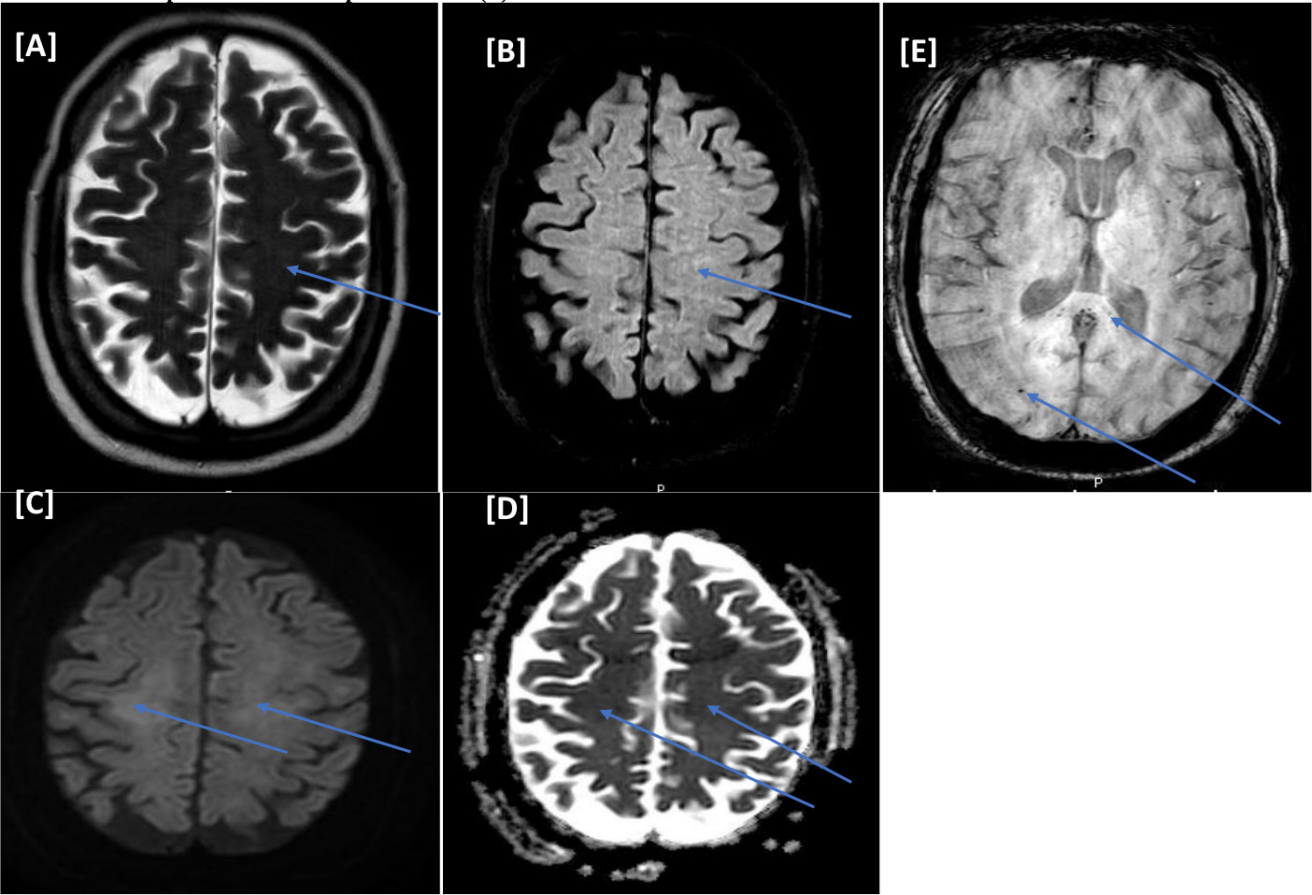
(Case III) [A–E images] Magnetic resonance imaging of the head. Bilateral centra semiovale white matter faint bright signal intensities on T1WI/FLAIR (A and B) with corresponding faint restriction on diffusion-weighted imaging series b1000/apparent diffusion coefficient (C and D). There are numerous variable-sized foci of blooming artifacts (microbleeds) on susceptibility-weighted images at the subcortical and splenium of the corpus callosum (E).
